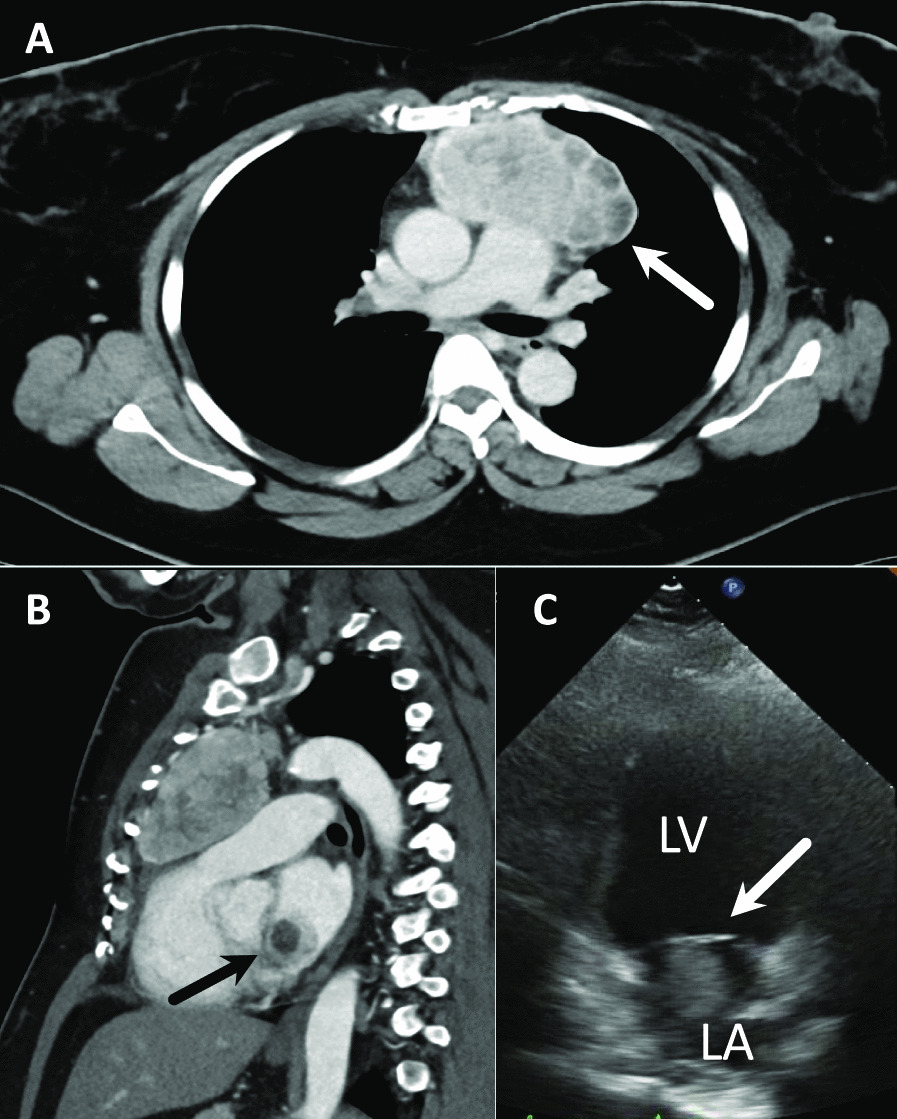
A Chest computed tomography showing a 100 × 90 mm anterior mediastinal mass with heterogeneous contrast (arrows). B Sagittal-view computed tomography. A 30 × 30-mm mass is identified in the left atrium (arrows). C Transthoracic echocardiography showing a mobile mass on the mitral valve. *LV* left ventricular, *LA* left atrium
